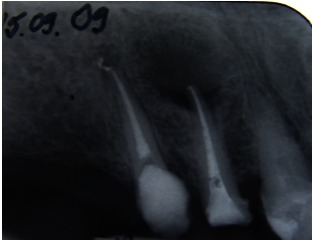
Fig. 212 postoperative radiograph
